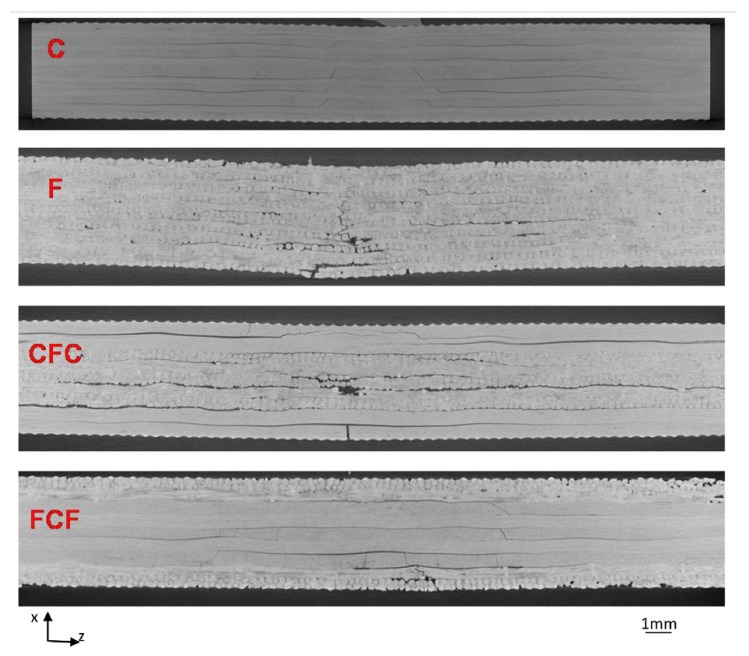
The computed tomography of different flax, carbon, and flax/carbon hybrid laminates impacted at 10 Joules [36] (Reproduction permission obtained).
